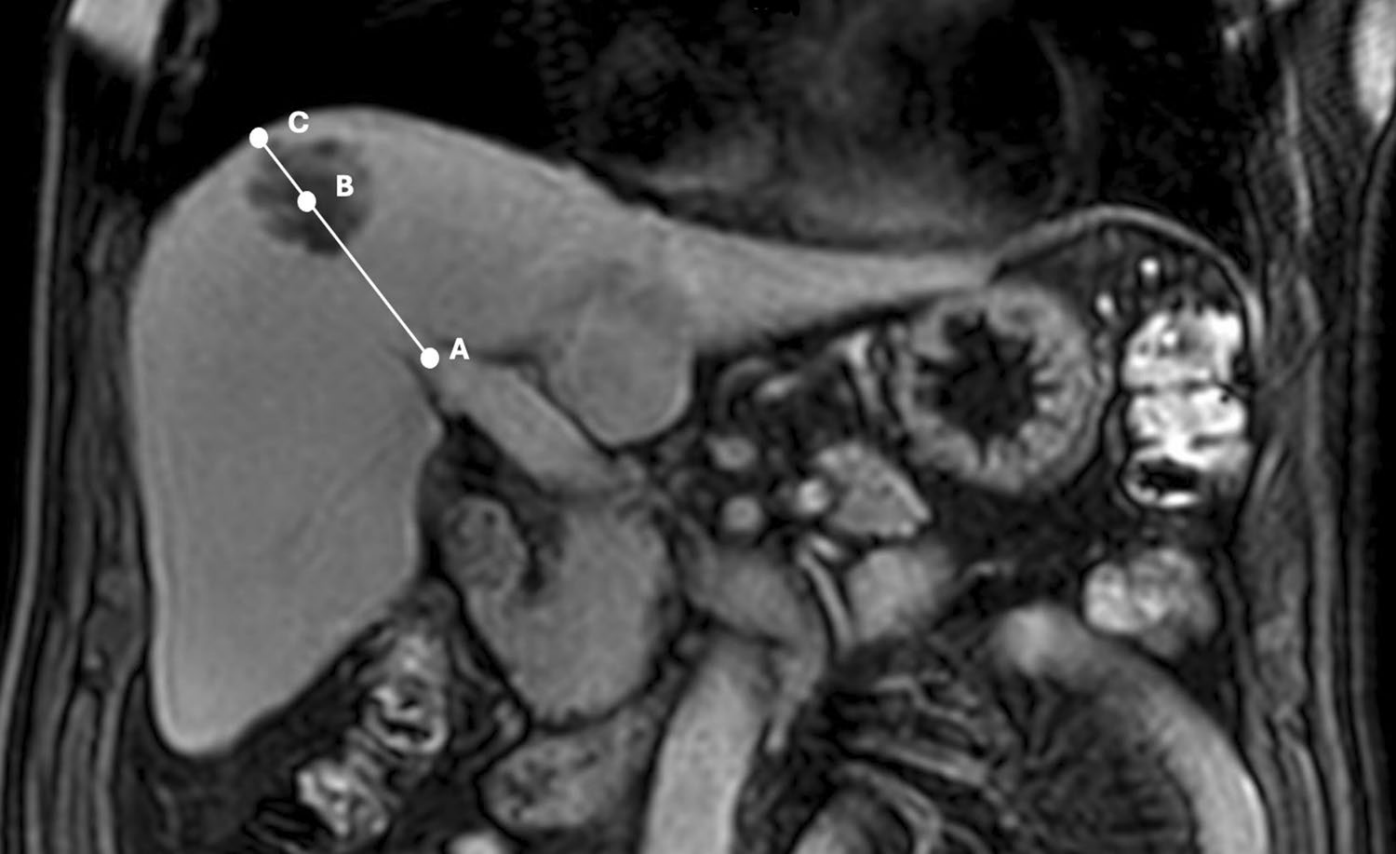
A late venous phase contrast-enhanced coronal T1 weigheted MR image. HCC location coefficient = distance from the bifurcation of the portal vein divided by the diameter of the whole liver on the same line (AB/AC). *MR* Magnetic resonance
